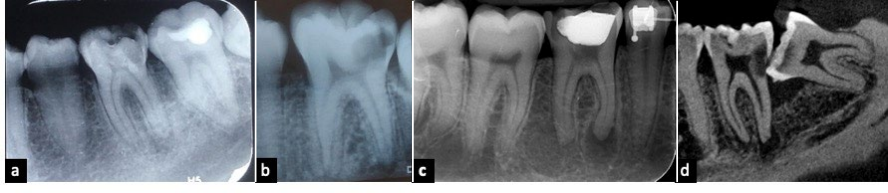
a) Radiografía periapical, diente 36; b) Radiografía periapical, diente 36; c) Radiografía periapical, diente 46; d) Imagen de CT, en corte sagital de diente 37. Se evidencian cambios en el aspecto de los tejidos periapicales de dientes con caries extensa o recurrente a restauración.
